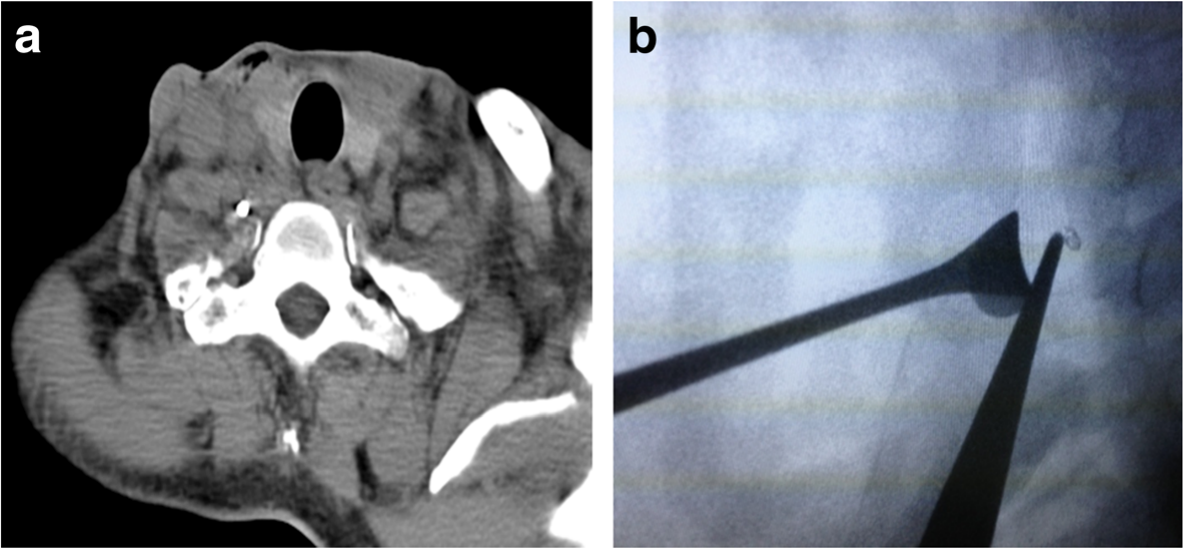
Images of the foreign body. (a) Plain computed tomography without contrast revealed a high density lesion impacted in the prevertebral space and its relationship with the carotid sheath and transverse process of the vertebral body. (b) X-ray image intensifier showed a radiopaque foreign body medial to the tip of the smooth pick-up, lateral to intervertebral discs of C7 and C8. A vein retractor holds the internal jugular vein posteriorly.
